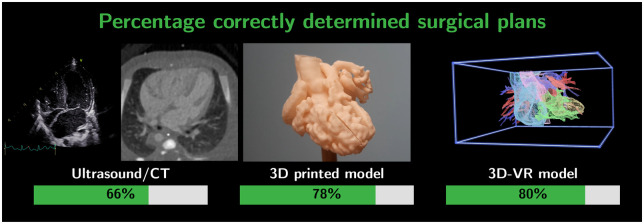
Number of surgical plans corresponding to the actual performed procedure, based on ultrasound and CT images (conventional 2D imaging), 3D printed models, and the 3D-VR models (left to right).
